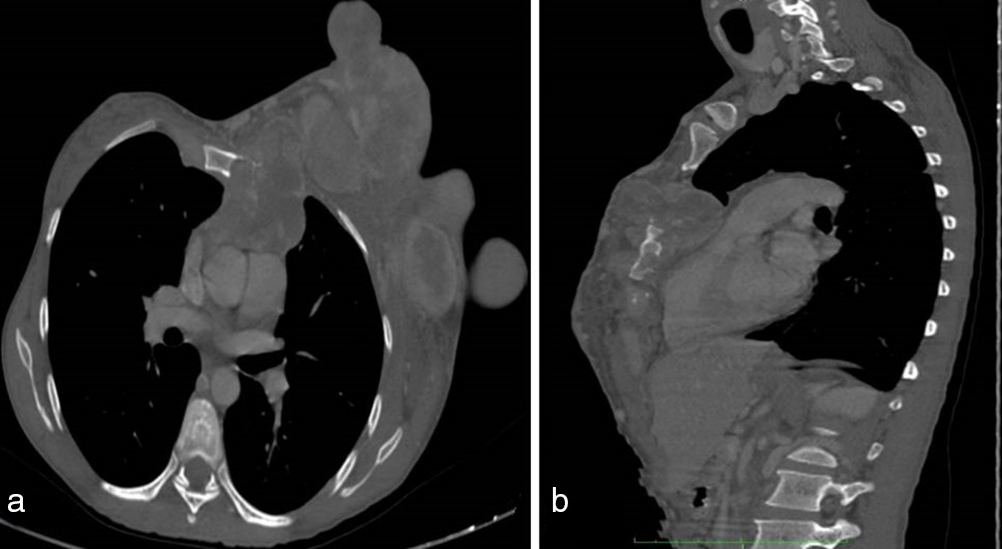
CT scan (a) axial and (b) reformatted sagittal bone window images showing erosion of the sternum.
